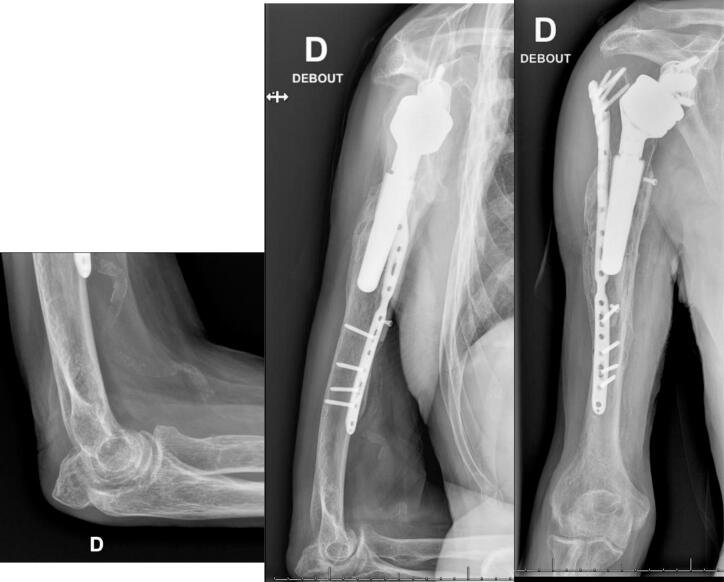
X-rays two years after the operation showing fracture consolidation with graft incorporation. Olecranon fracture showing radiological signs of union.
